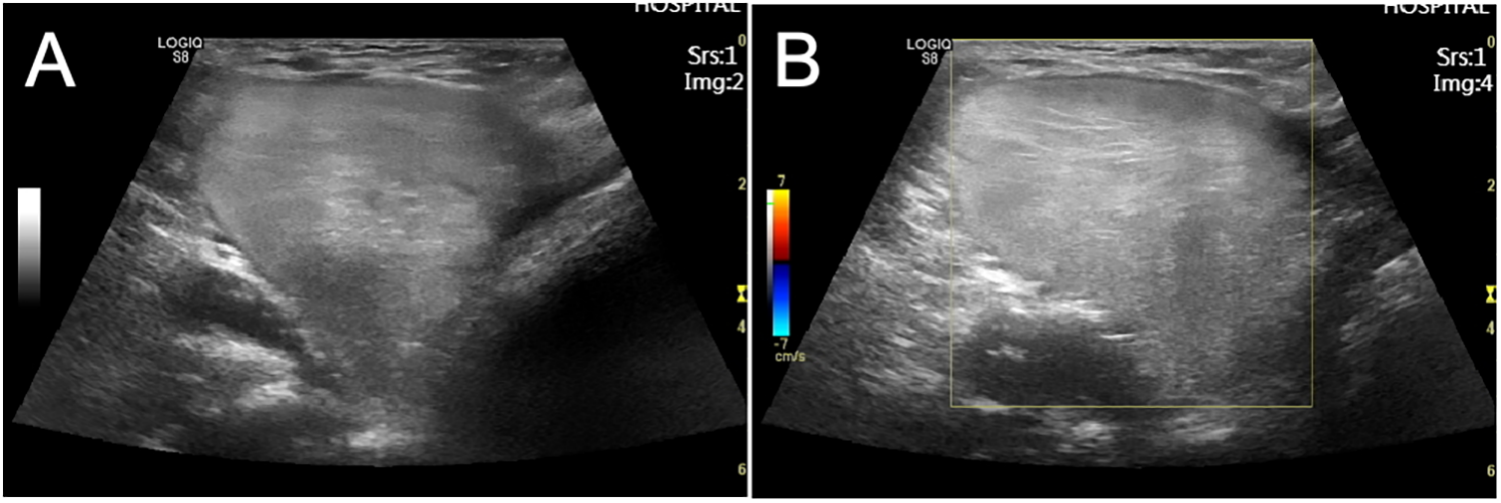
(A,B) In the right anterior chest wall near the axillary line, the mass measured 5.1 cm × 4.3 cm × 4.8 cm, with an irregular shape, non-uniform internal echo, and a small amount of blood flow signal on color Doppler ultrasound, suggesting a mesial tissue tumor.
